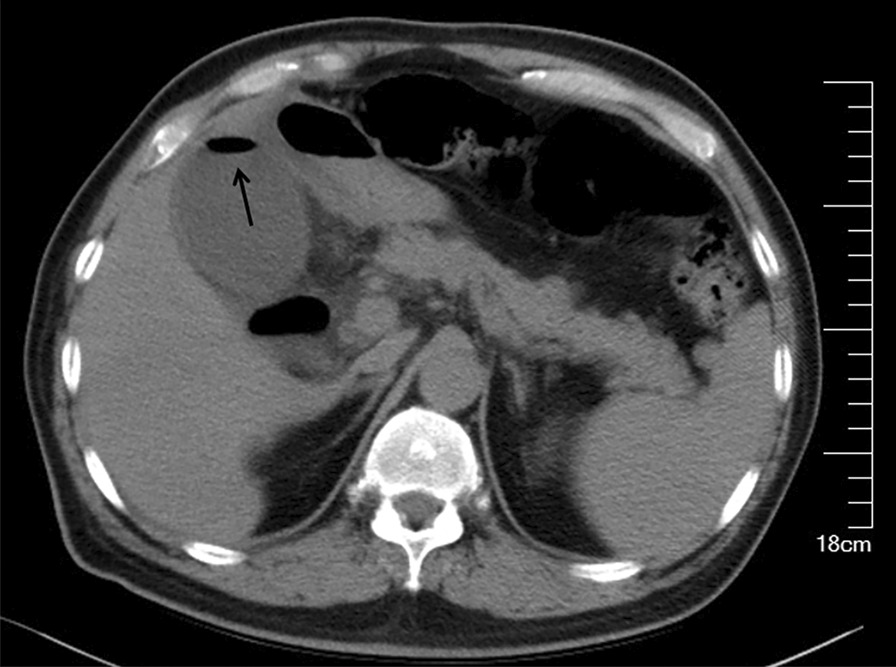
A 59-year-old male with gas within the gallbladder lumen. A nonenhanced CT showed gas (arrow) within the gallbladder lumen. The patient underwent PC on the same day, and the bile was purulent
